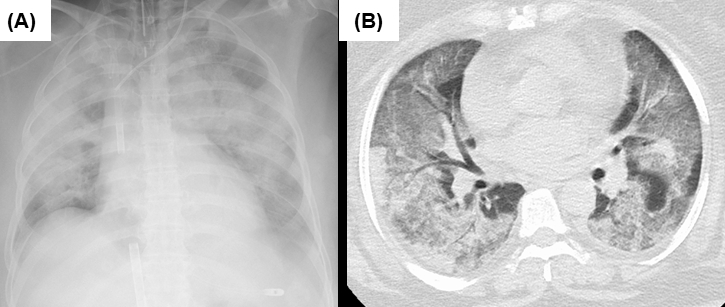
Chest radiograph and computed tomography on admission. (A) Chest radiograph right after cannulation of ECMO, which showed extensive ground-glass and infiltrative shadows in bilateral lung fields. A drainage cannula was placed in the inferior vena cava and a return cannula was placed in the superior vena cava. (B) Chest computed tomography on admission showed extensive ground-glass shadows in both lungs with partially overlapping infiltrative shadows. Interlobular septal wall thickening and traction bronchiectasis are unremarkable.
